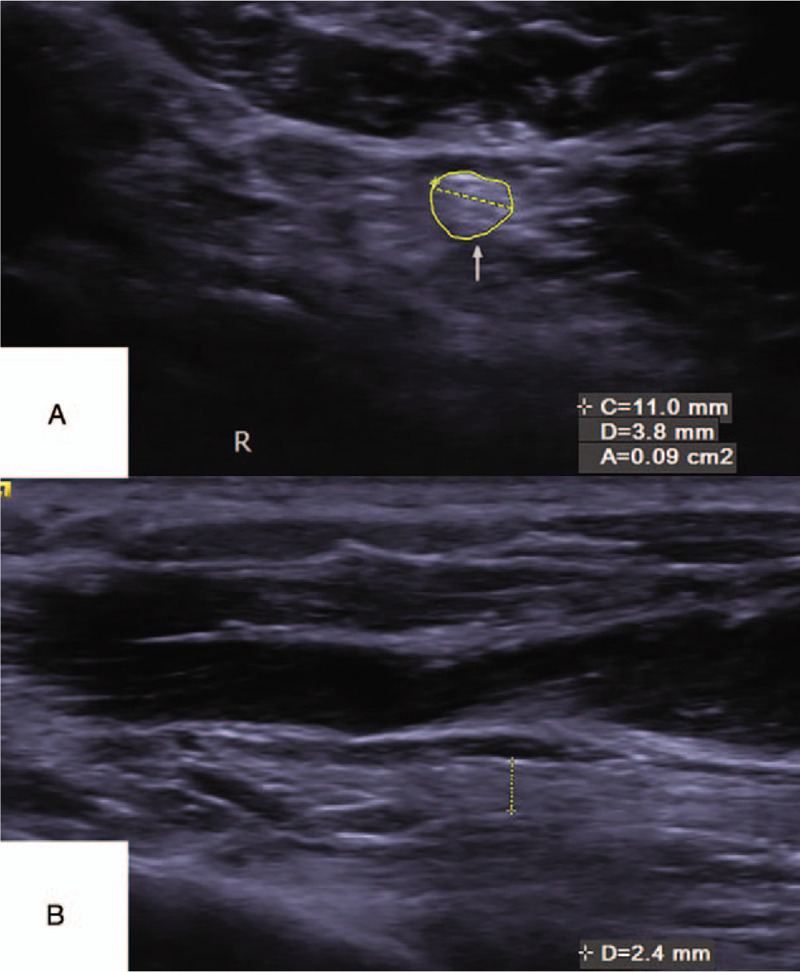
The results of musculoskeletal ultrasonography after 2 mo conservative treatment. The CSA of right LPN was 9 mm^2^, the length diameter on the cross section of the LPN was 3.8 mm (A), and the thickness of the longitudinal section was 2.4 mm (B). CSA = cross sectional area, LPA = lateral plantar nerve.
